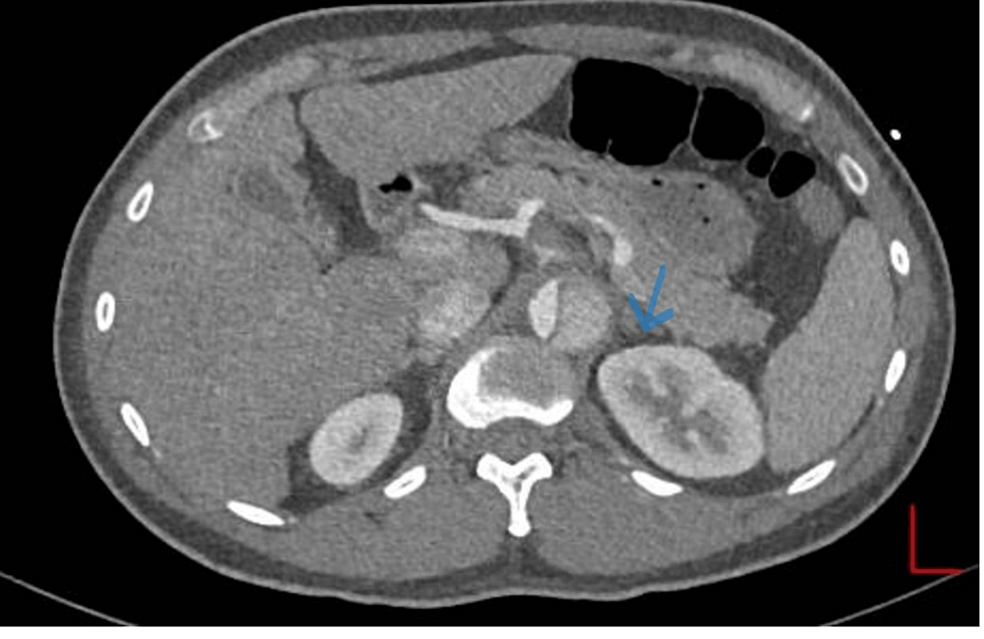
CT thoracic and abdominal aorta was performed two days later. Exhibiting a lack of enhancement of the adrenal gland in keeping with ischemia of the left adrenal gland (blue arrow).
